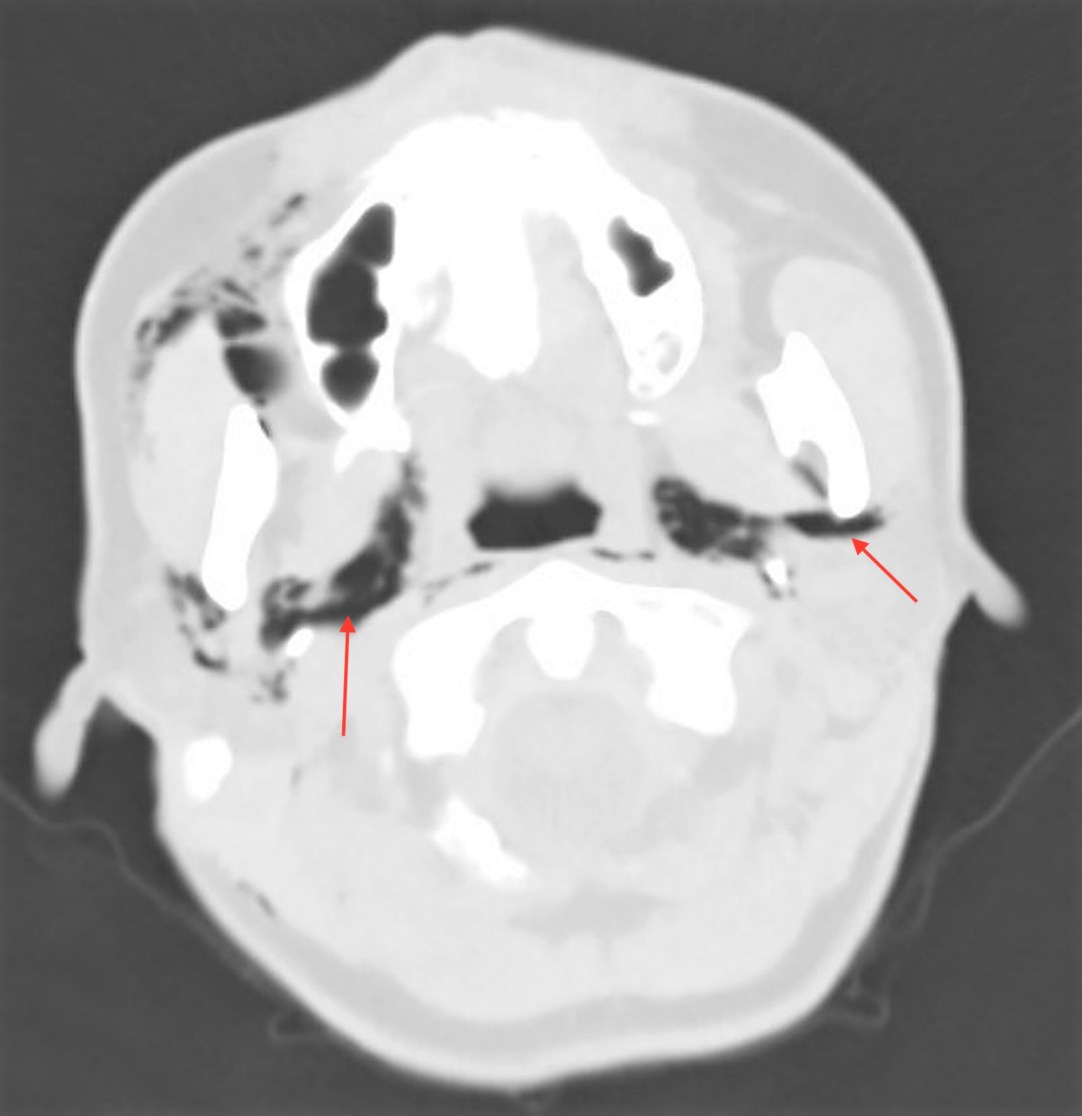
Axial CT image of facial soft tissues with air in the carotid and retropharyngeal space.
